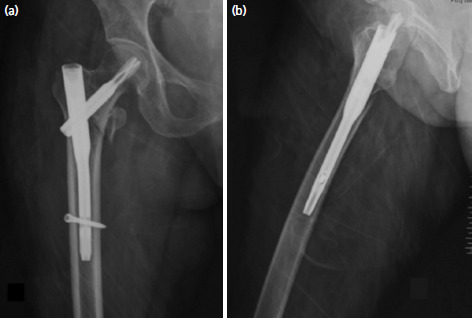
(a) Antero-posterior (AP) radiograph showing good reduction and implant positioning using short cephalo-medullary nail, (b) lateral radiograph of the same patient.
